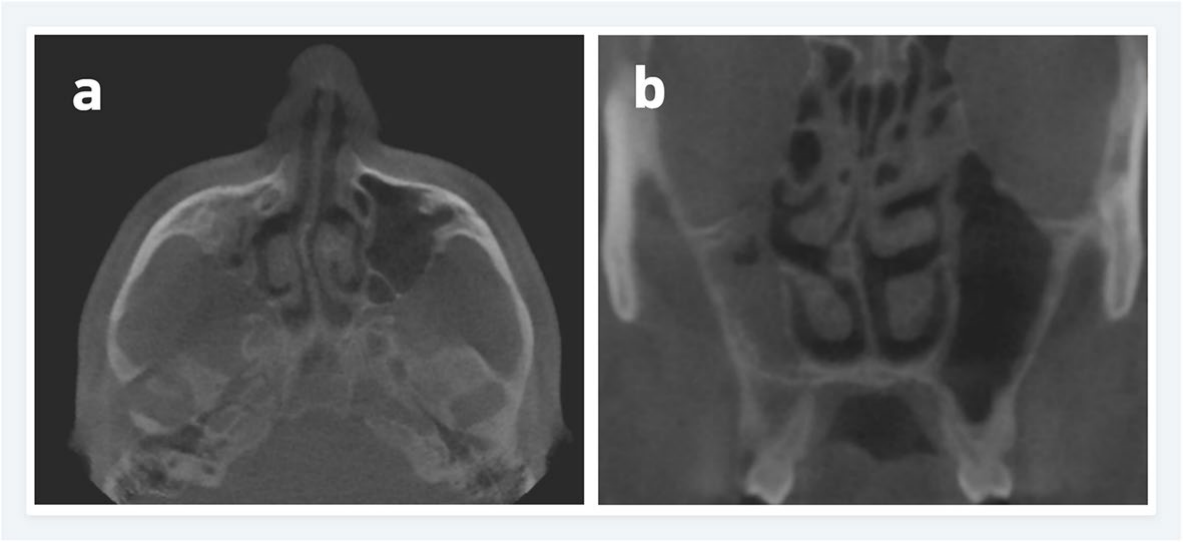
CBCT images of the nasal cavity and paranasal sinuses. Axial (a) and coronal (b) planes showing right side nasal septum deviation with septal spur (b) and maxillary sinus hypoplasia on the right side
